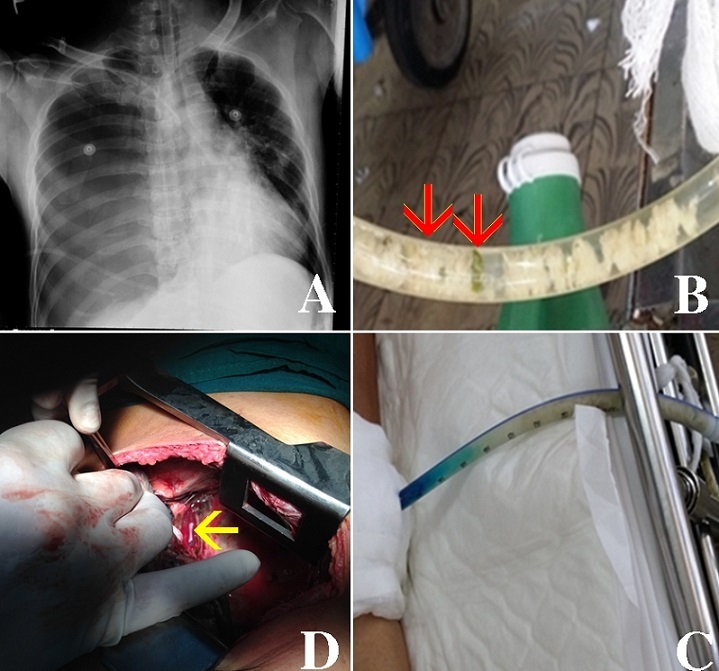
Boerhaave’s syndrome with food particles in chest tube: (A) chest x-ray showed right-sided tension pneumothorax; (B) rice and green onions (red arrows) appeared in the chest tube; (C) methylene blue which was swallowed came out through the chest tube; (D) a part of the nasogastric tube (yellow arrow) was found during the thoracotomy process
